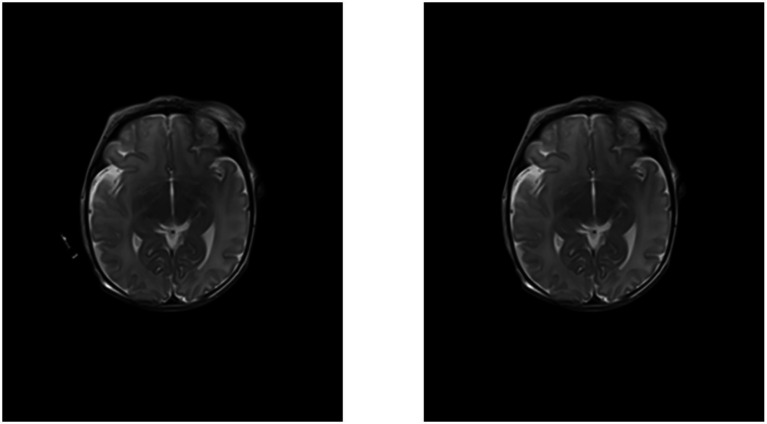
Axial slice of a T2-weighted MRI scan before (left) and after (right) artifact removal.
